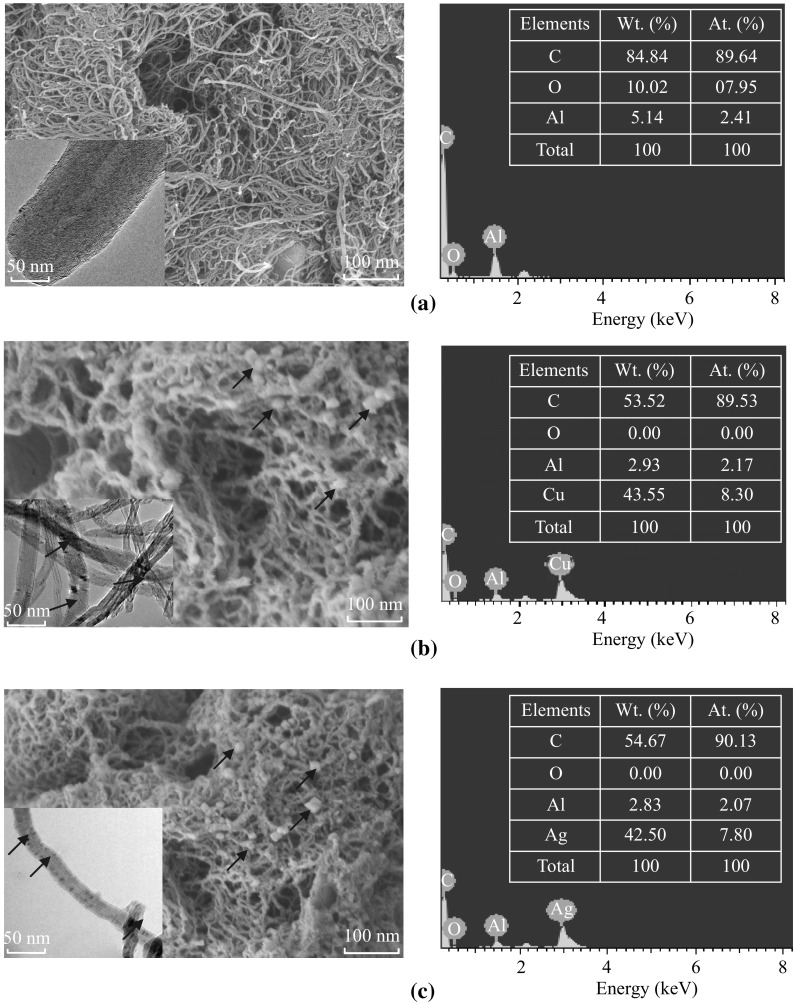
FESEM images and corresponding EDX spectra of MWCNTs (a), Cu-MWCNTs (b), and Ag-MWCNTs (c). The corresponding TEM images (*inset*) shows the smaller MNPs trapped (embedded) endohedrally along the inner surface of MWCNTs, while the exohedrally functionalized MNPs were removed when dispersed in ethanol and acetone mixture in ultrasonic bath. (Color figure online)
